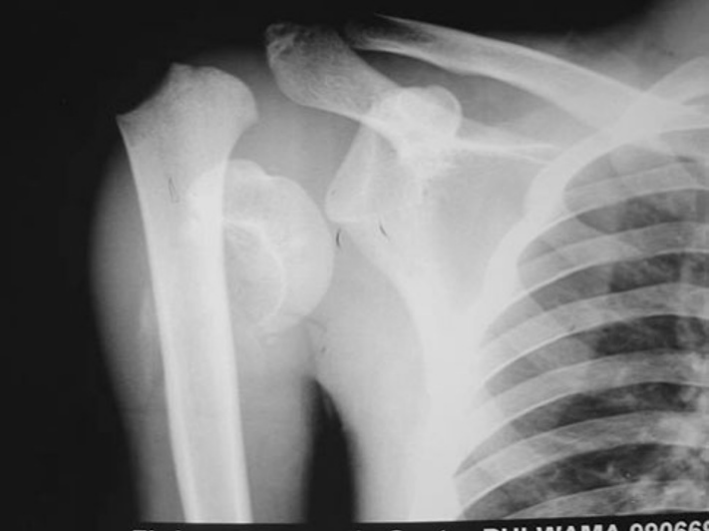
Radiograph of the affected shoulder at 4 weeks
